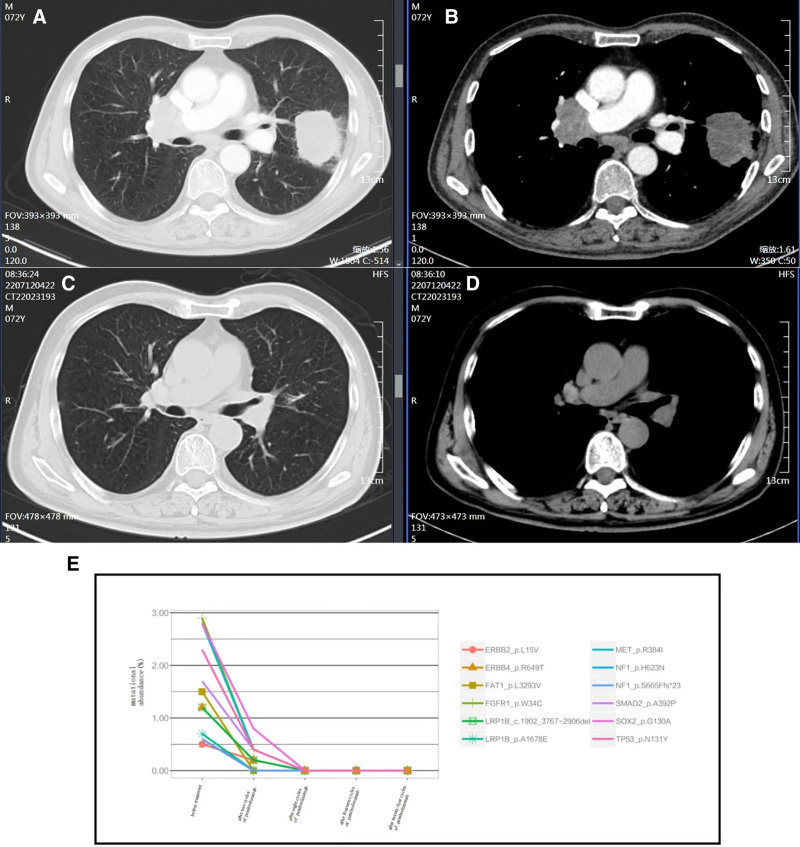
Computed tomography images before and after the pembrolizumab monotherapy. Before the treatment, there were bilateral multiple pulmonary nodules with a mass in the left upper lobe (arrow) (A) and enlarged lymph nodes in the mediastinum and right hilar area (arrows) (B). After the treatment for 8 cycles, the left lung mass disappeared (C), and the lymph nodes in the mediastinum and right hilar area had significantly reduced sizes (D). Circulating tumor DNA abundance in the blood samples decreased significantly after the 2 cycles of the pembrolizumab monotherapy (E).
